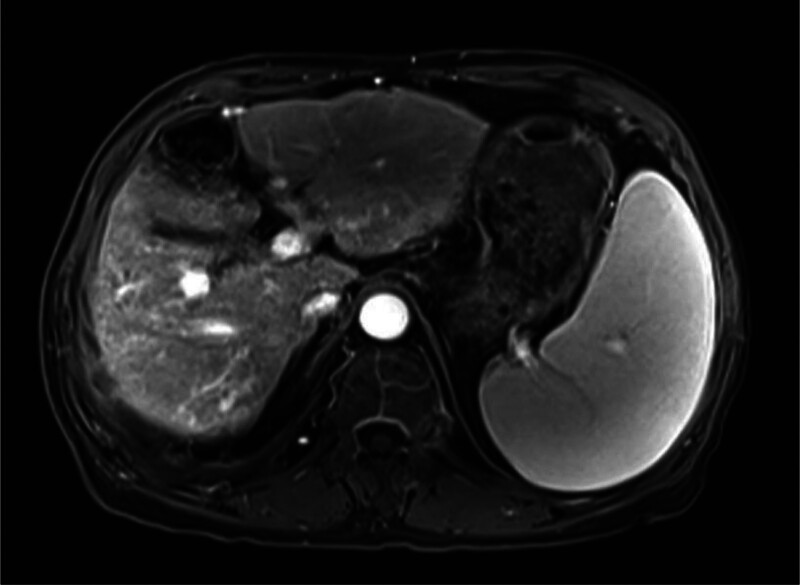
Upper abdominal MRI showed the gallbladder was slightly larger, which might have been caused by liver cirrhosis; kidney atrophy and small cysts were also observed. MRI = magnetic resonance imaging.
